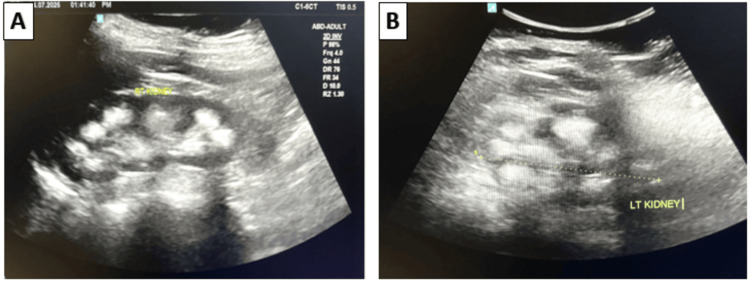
Ultrasonography (USG) of lower abdomen USG of lower abdomen showing multiple echogenic foci in the medullary renal parenchyma of the left (A) and right kidney (B).
